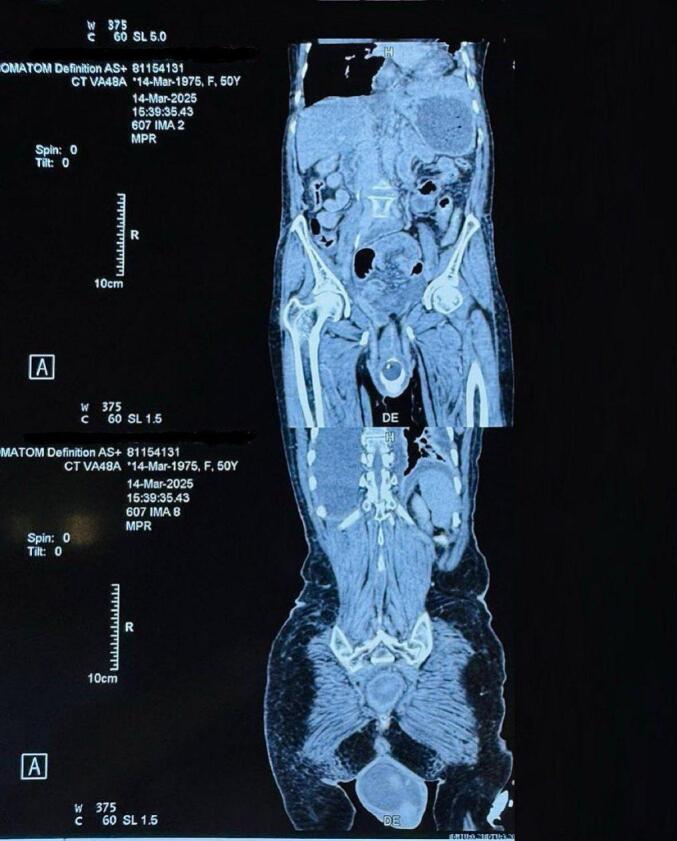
MRI image showing rectal perforation and uterine prolapse.
